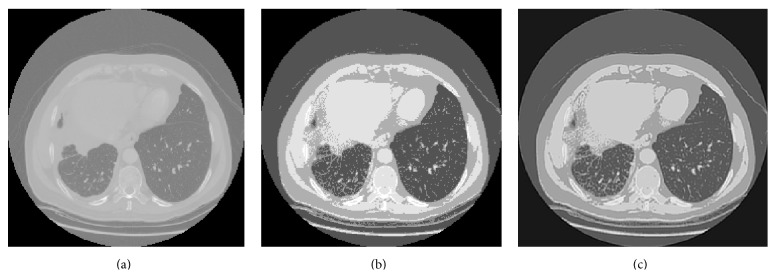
(a) The lung CT image; (b) the histogram equalized image; (c) the Weiner filtered output image.
